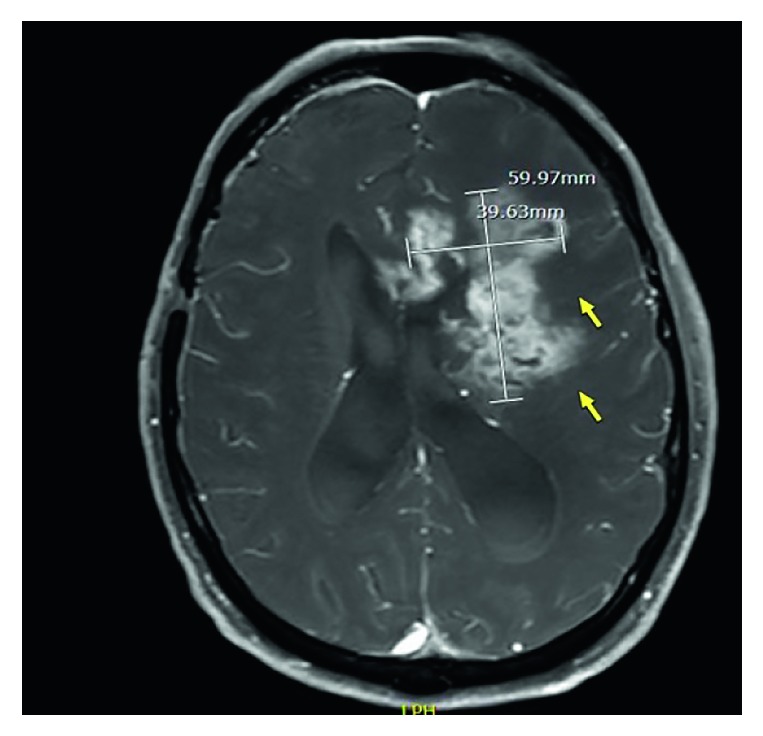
MRI brain axial view demonstrating multiple enhancing masses coalescing into a larger mass with surrounding edema resulting in midline shift involving anterior corpus callosum.
